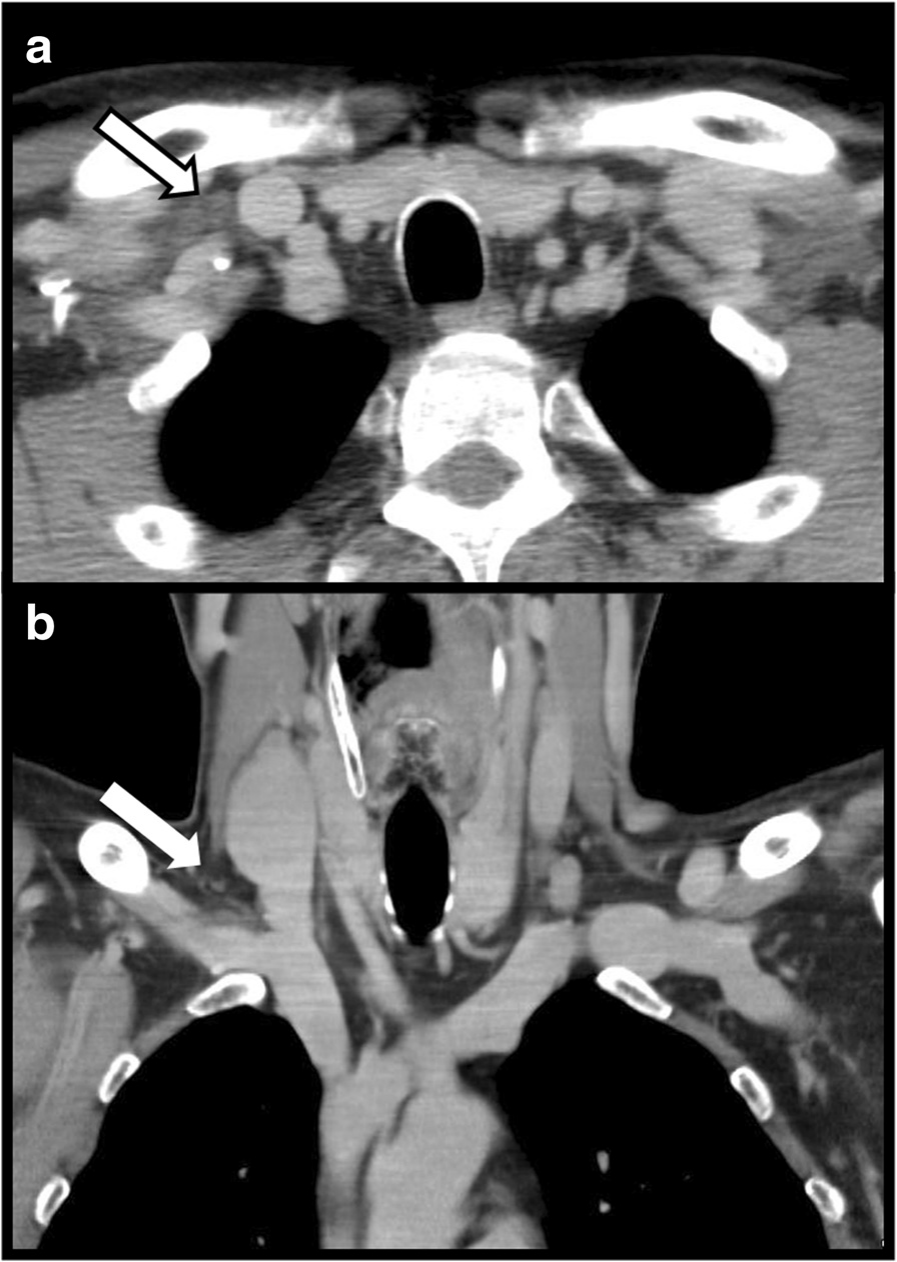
A 54 year old male with cancer of the tongue. Contrast enhanced computed tomography in the axial (a) and coronal (b) plane. Dendritic configuration of the right lymphatic duct (arrows)
